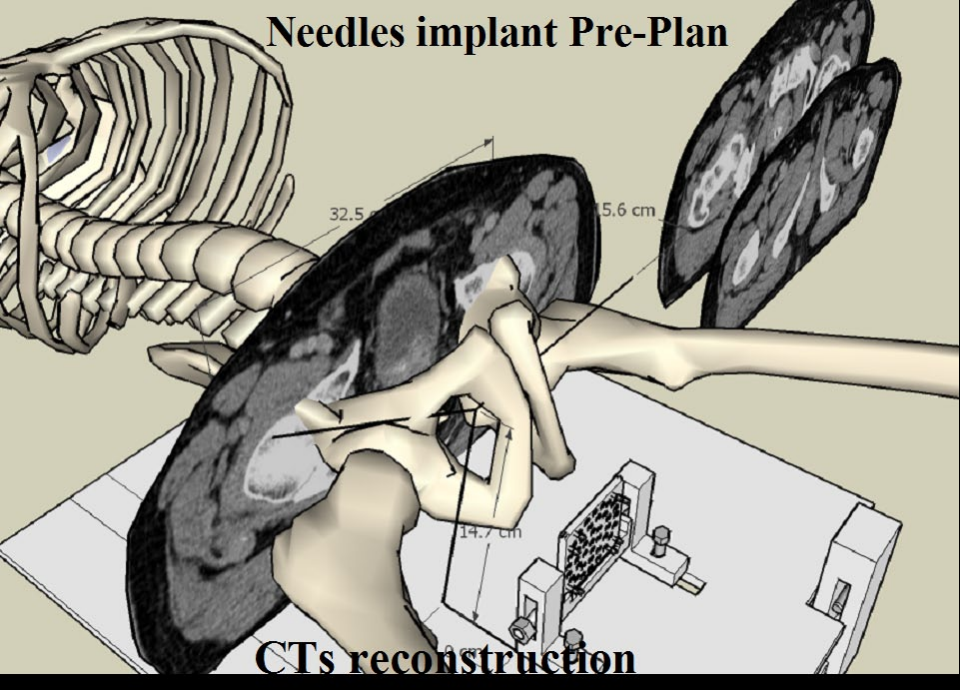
This procedure is to arrange CT images slice by slice reconstructed to a preplan system and to avoid needle puncture of the urethra. All needle insertions are conducted with equal geometry distribution between the needles to cover the prostate gland with incline insertion to avoid bone obstruction.
